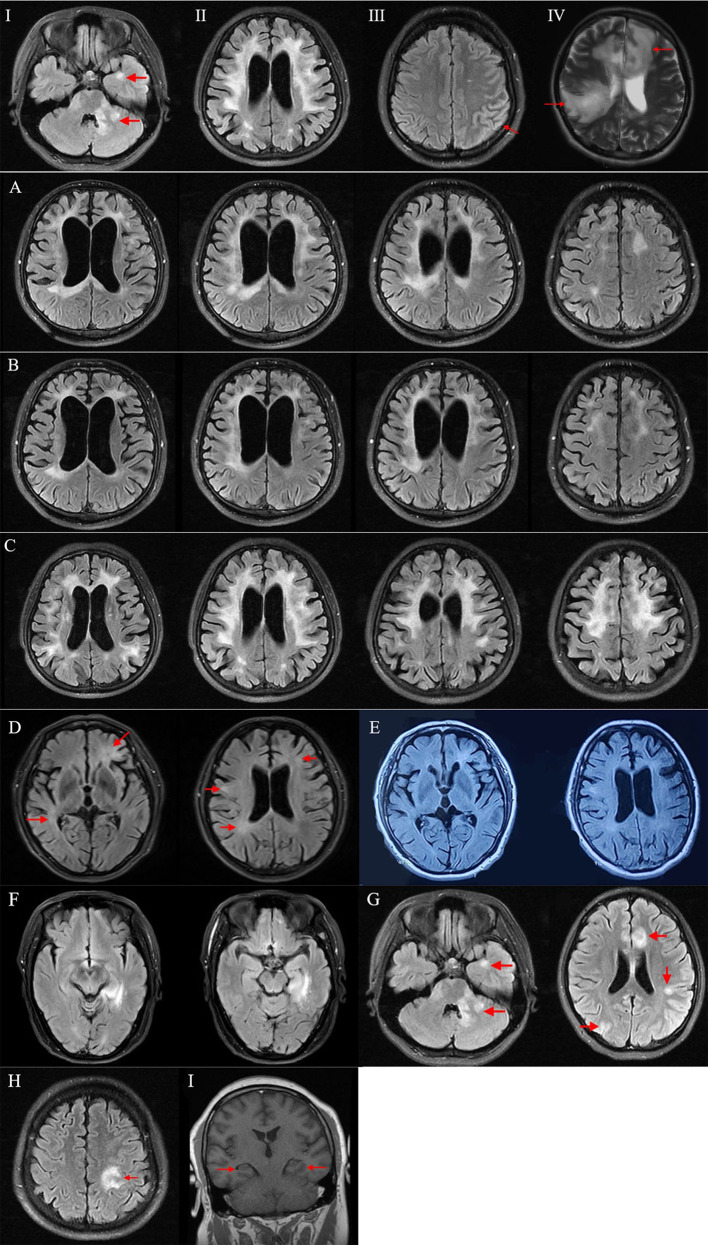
Brain MRI in MOG-E patients. (I–IV): Representative images of four brain MRI patterns in MOG-E. (I) Multifocal hazy/poorly marginated lesions; (II) leukodystrophy-like pattern; (III) cortical encephalitis with leptomeningeal enhancement/brain atrophy; (IV) tumefactive demyelinating lesion. (A–F): Brain MRI in chronically progressive MOG-E. (A) Brain MRI of patient 10 in 2018 showed leukodystrophy-like white matter change with whole-brain atrophy. (B) Brain MRI of patient 10 in 2019 showed slight improvement of white matter change on MRI. (C) Brain MRI of patient 11 showed leukodystrophy-like pattern with whole-brain atrophy. (D) Brain MRI of patient 12 in 2020 showed multifocal hazy and poorly marginated lesions. (E) Brain MRI of patient 12 in 2021 showed no prominent improvement of lesions. (F) Brain MRI of patient 13 showed a lesion in the left hippocampus and parahippocampal gyrus. (G–I): Brain MRI abnormalities in patients with overlapping MOG-ab and NMDAR-ab. (G) Patient 5 showed multifocal poorly marginated lesions in cortical gray matter, subcortical white matter, and midline structures on MRI. (H) Patient 4 showed cortical and subcortical lesions in the left frontal and parietal lobes on MRI. (I) Patient 7 showed mild bilateral hippocampi atrophy without lesion on MRI. ab, antibodies; MOG-E, MOG-antibody-associated encephalitis.
